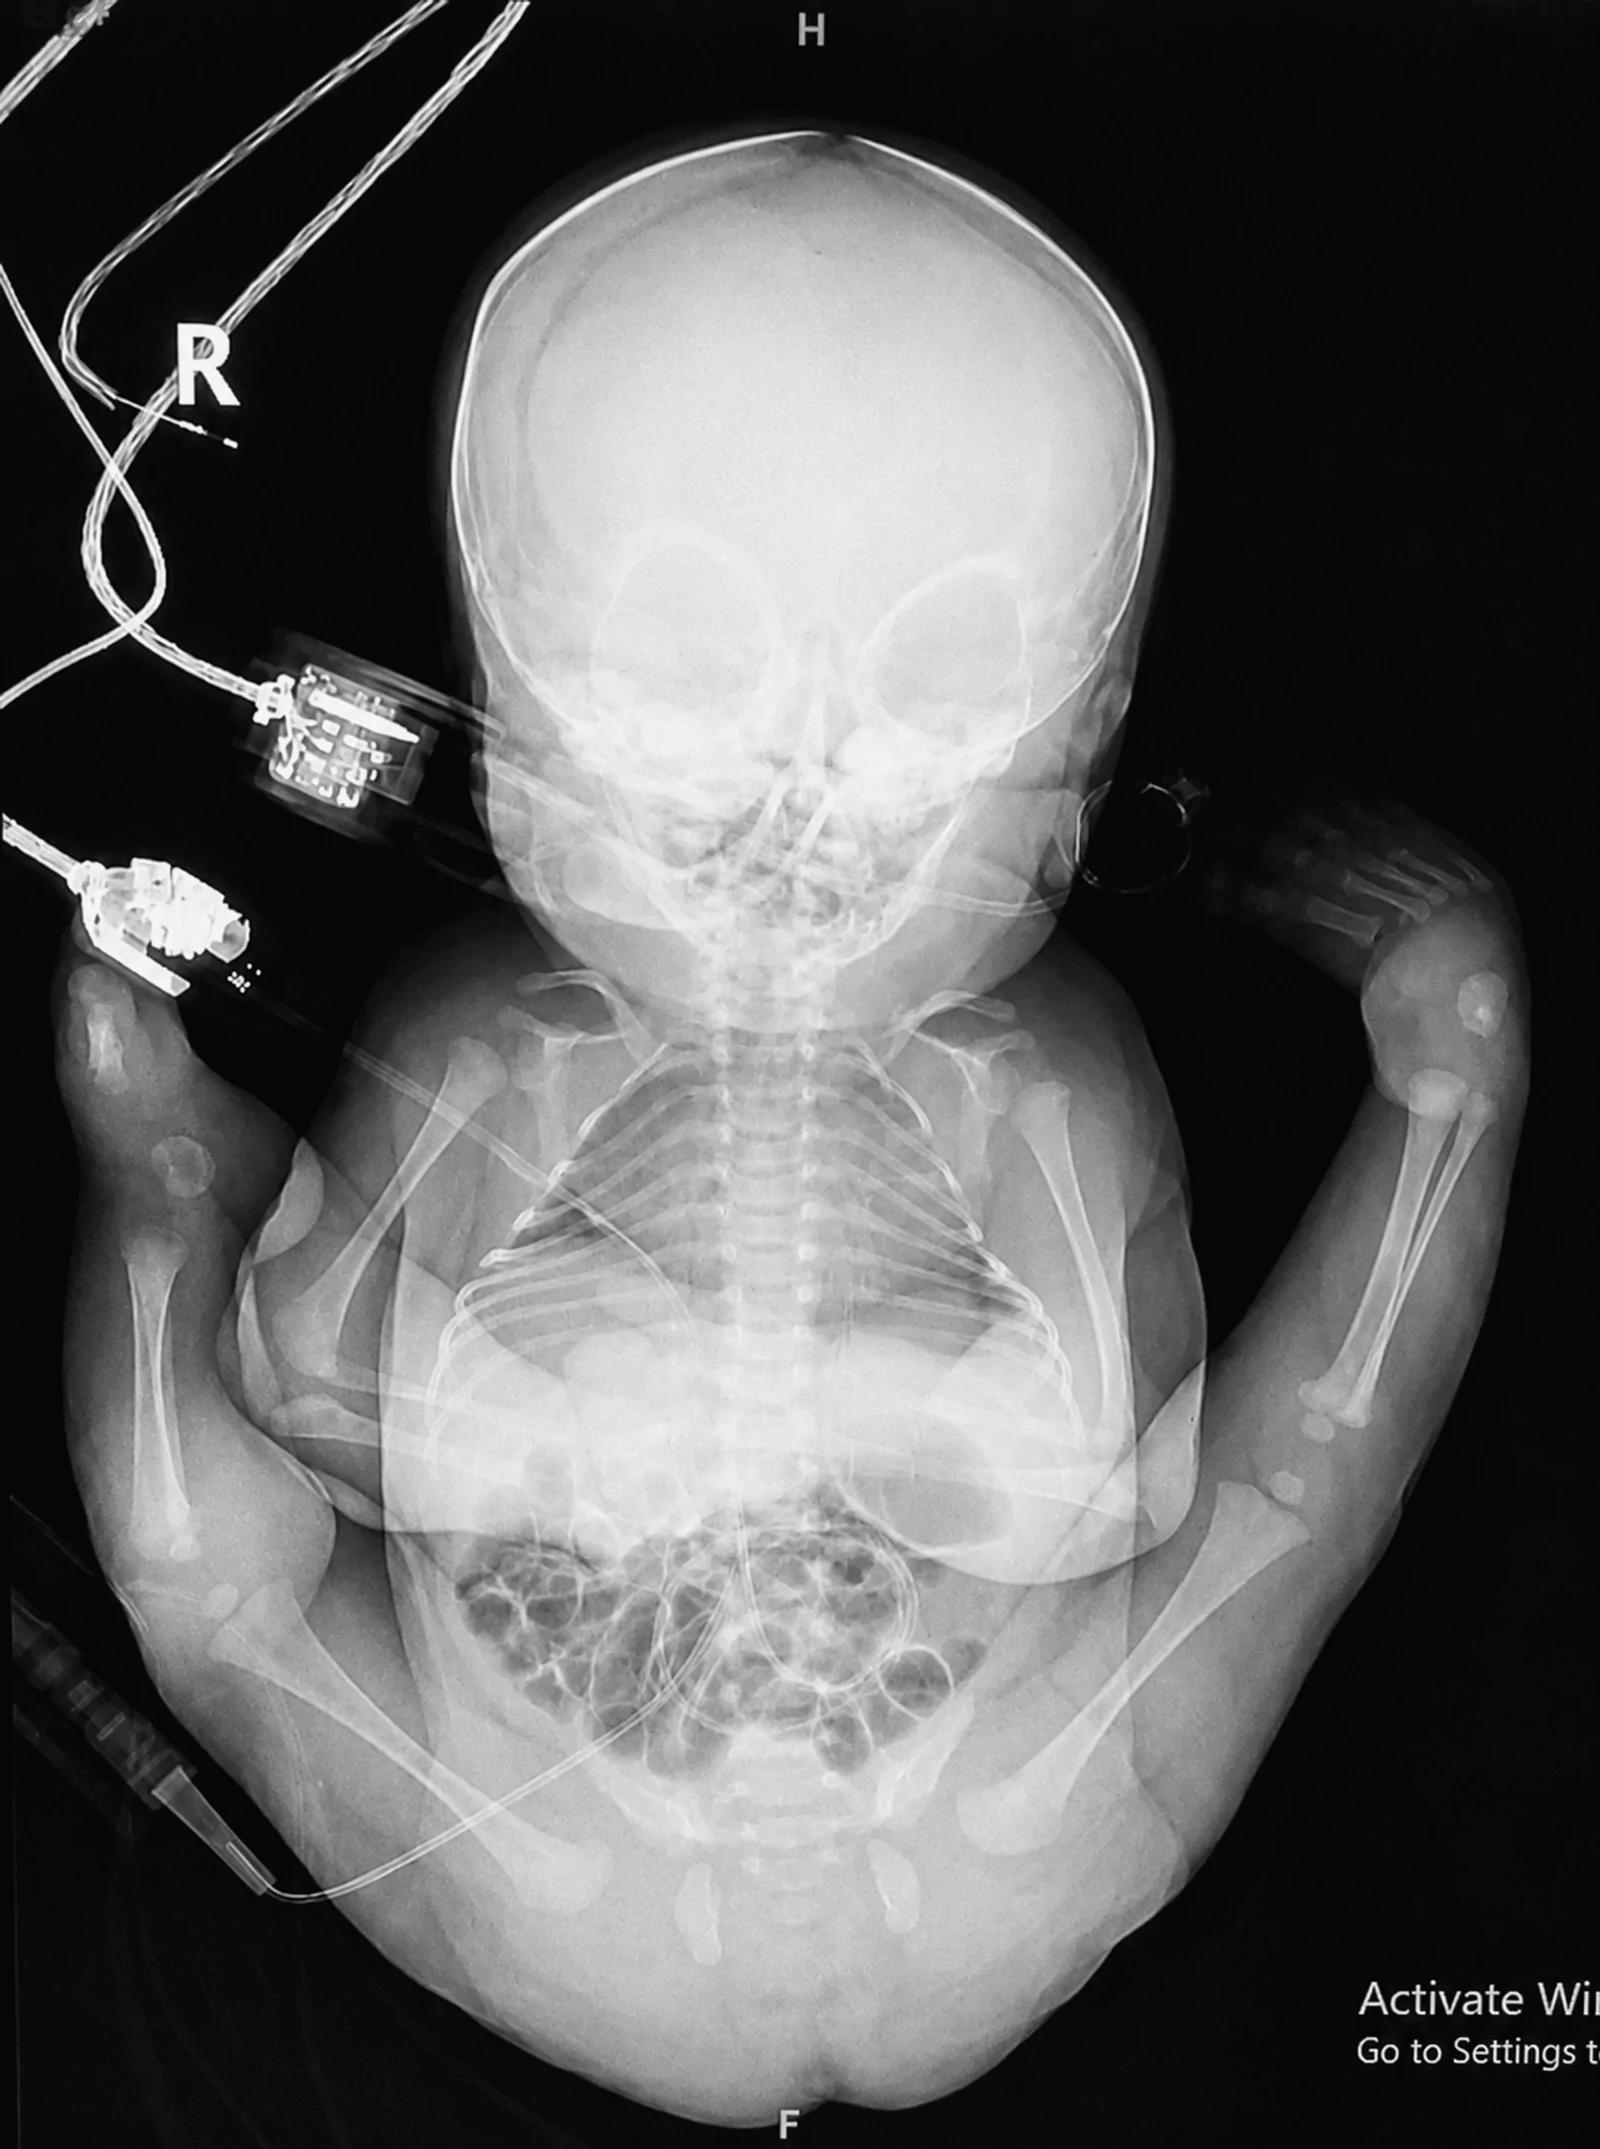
Preoperative whole-body anteroposterior radiograph of the neonate showing multiple congenital musculoskeletal deformities consistent with arthrogryposis multiplex congenita (AMC), including severe limb contractures and craniofacial abnormalities, indicative of a predicted difficult airway.
